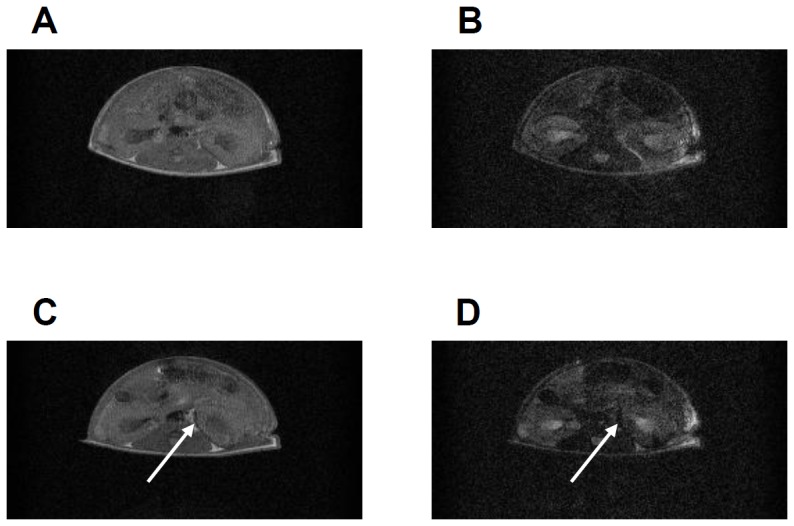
In vivo MRI of transplanted islets labeled with TMADM-03. A. T1-weighted images of unlabeled islets (1000 IEs) transplanted into the left kidney capsule. B. T2-weighted images of unlabeled islets (1000 IEs) transplanted into the left kidney capsule. C. T1-weighted images of islets (1000 IEs) that were labeled with TMADM-03 and transplanted into the left kidney capsule. D. T2-weighted images of islets (1000 IEs) that were labeled with TMADM-03 and transplanted into the left kidney capsule. The arrows show transplanted grafts.
